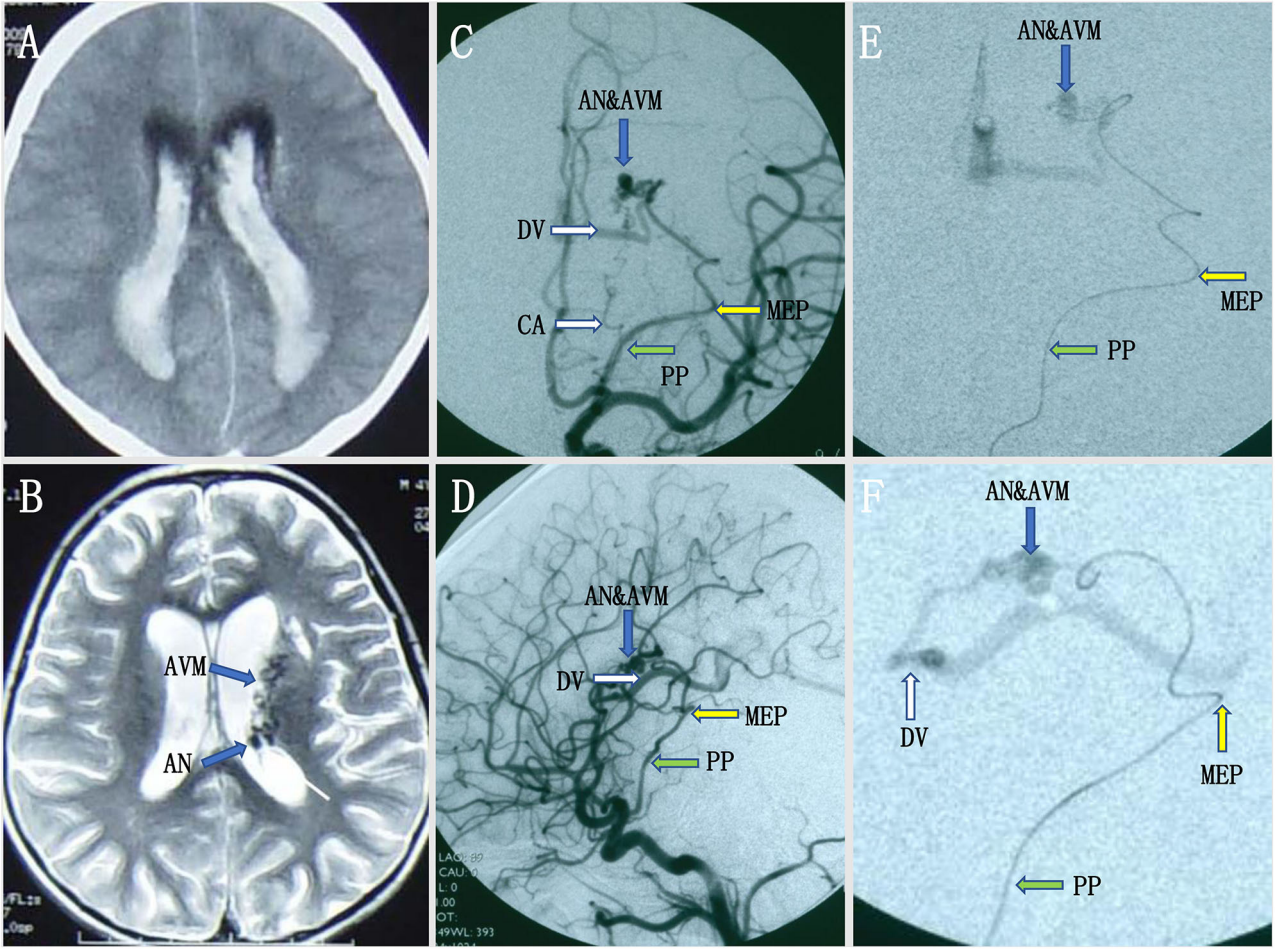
(A,B) Intraventricular hemorrhage was noted on initial computed tomography (CT), and subsequent magnetic resonance imaging (MRI) revealed multiple signal-void vascular structures in the lateral ventricle with enlarged aneurysmal structures. (C,D) Preoperative digital subtracted angiography (DSA) revealed a distal AChoA aneurysm associated with an intraventricular arteriovenous malformation (AVM) with deep venous drainage via an enlarged internal cerebral vein and the great cerebral vein of Galen into the straight sinus, visualized in the early DSA. (E,F) The ultra-selective DSA [anteroposterior (AP) view (E) and lateral view (F)] in the operation showed that the microcatheter had crossed the most external point (MEP) of the anterior choroidal artery (AChoA), and the microcatheter tip rested on the right point, as our planned onyx reflux point was distal to the MEP.
